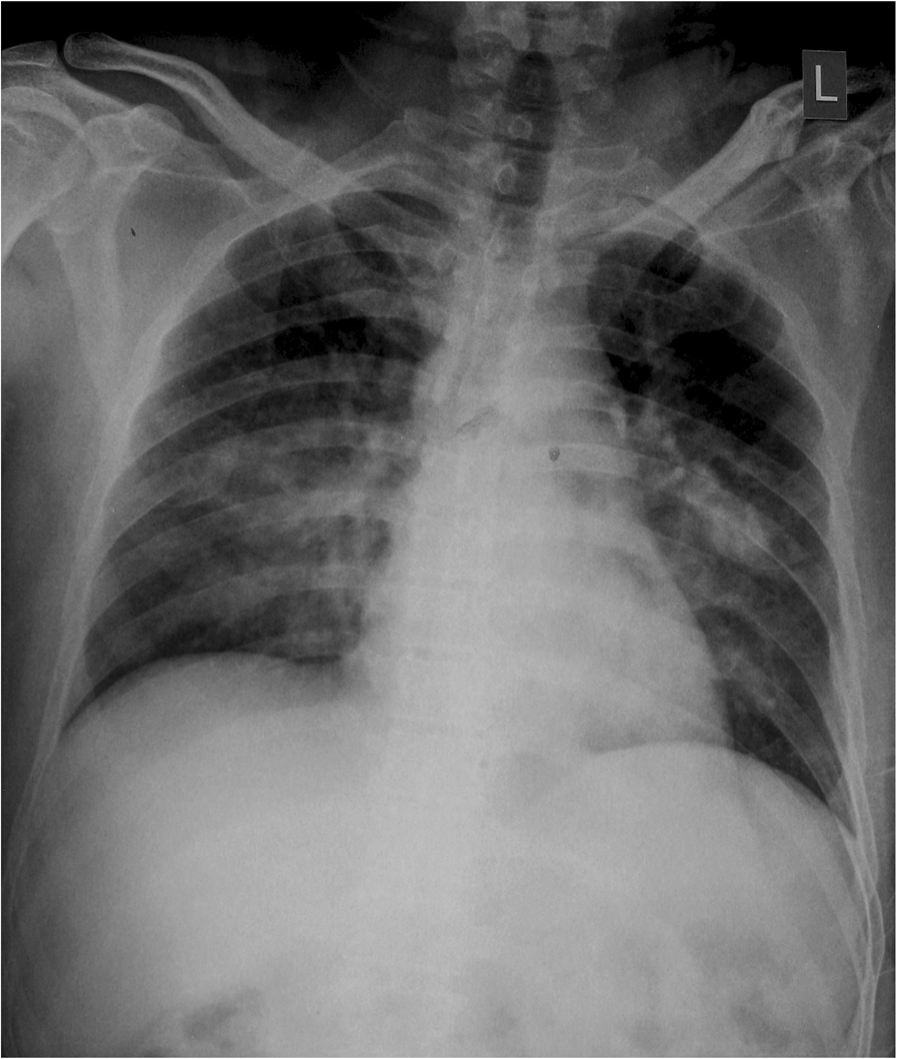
Chest radiograph showing bilateral non-homogenous fluffy infiltrates.
